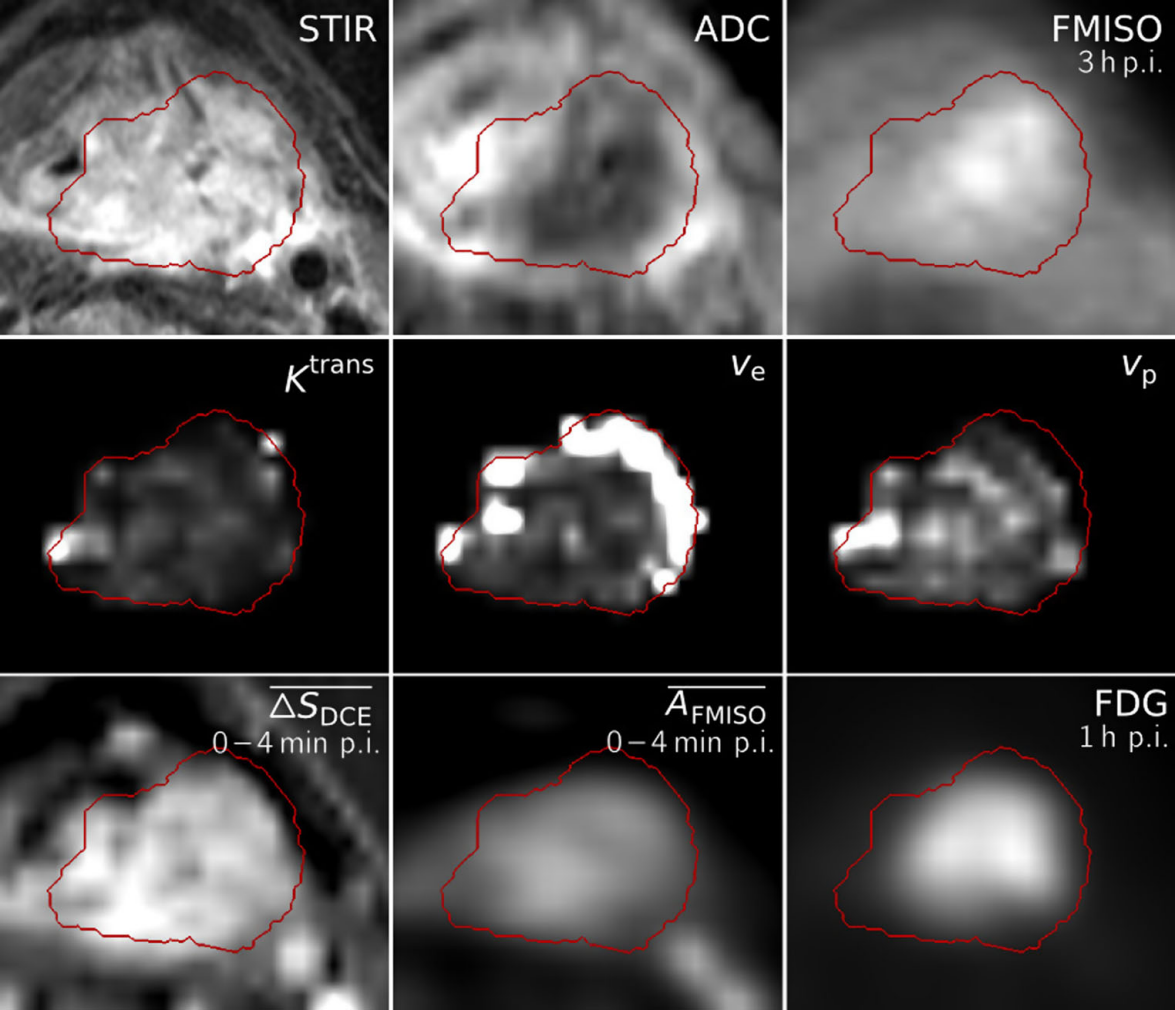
Dataset of Patient 11, showing transversal slices of the anatomical T2-weighted image (STIR), ADC map, FMISO image at 3 h p.i., the DCE-derived maps *K*
